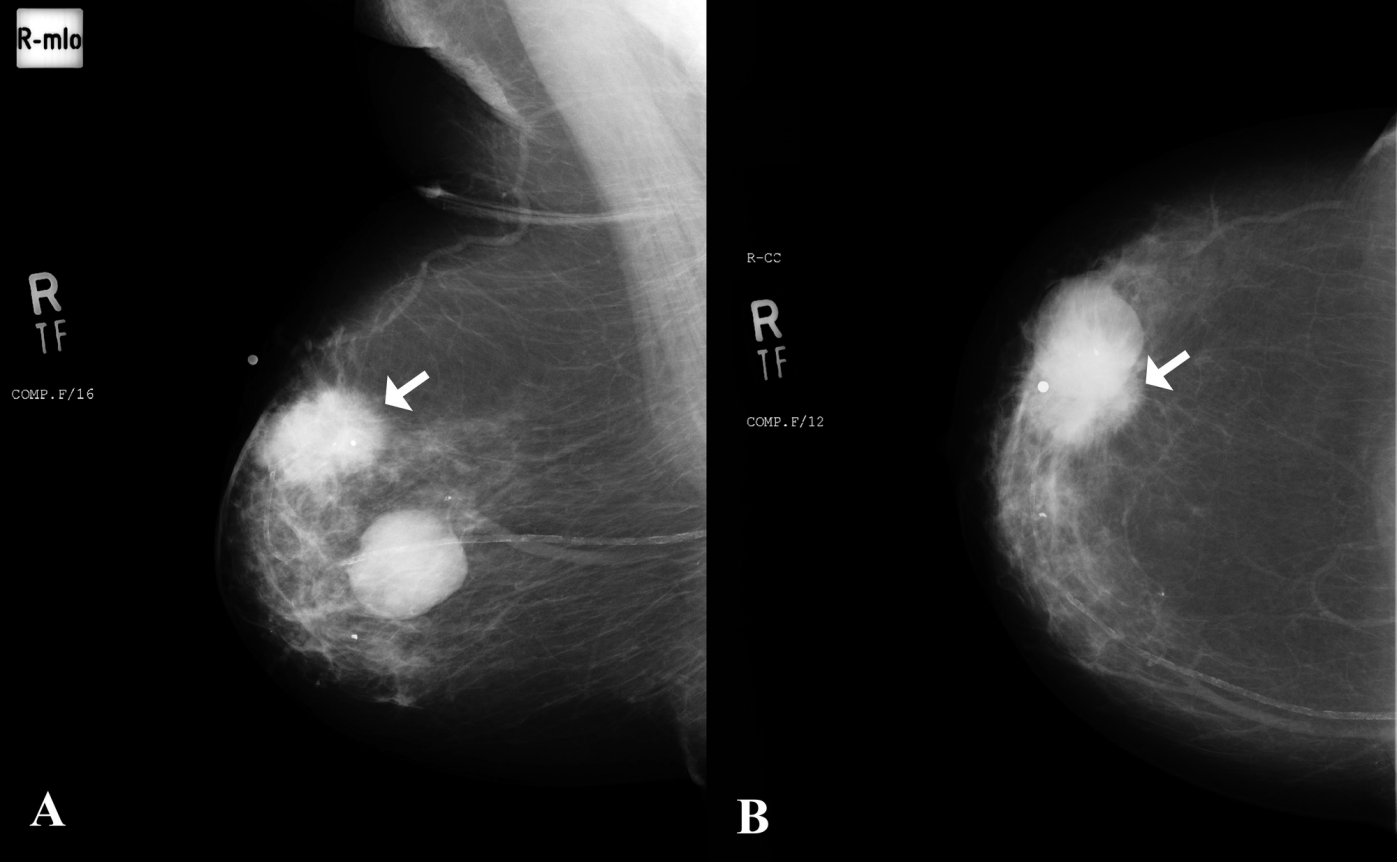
A) Mediolateral oblique and B) craniocaudal views of mammogram in an 82-year-old female Large lesion with spiculated margins in the upper outer quadrant of right breast consistent with a neoplastic lesion (arrows). A well-defined rounded density adjacent to this suspicious lesion was a cyst as correlated on ultrasound.
